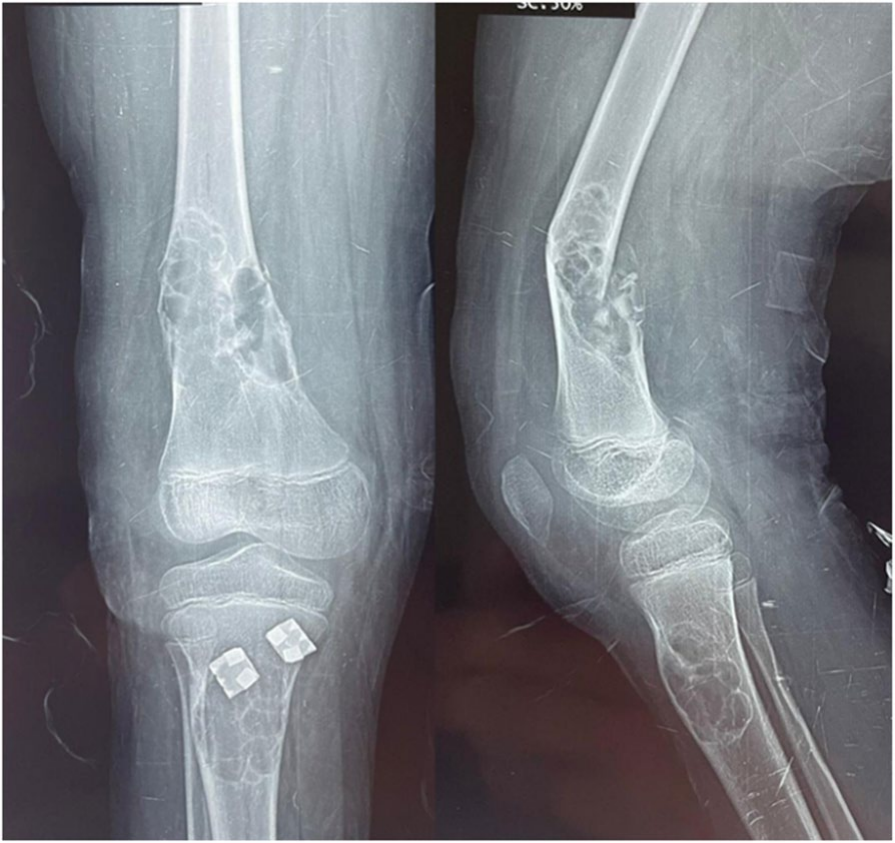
Showing an X-ray of the right femur fracture over the NOFs. A lesion in the proximal tibia is also seen
